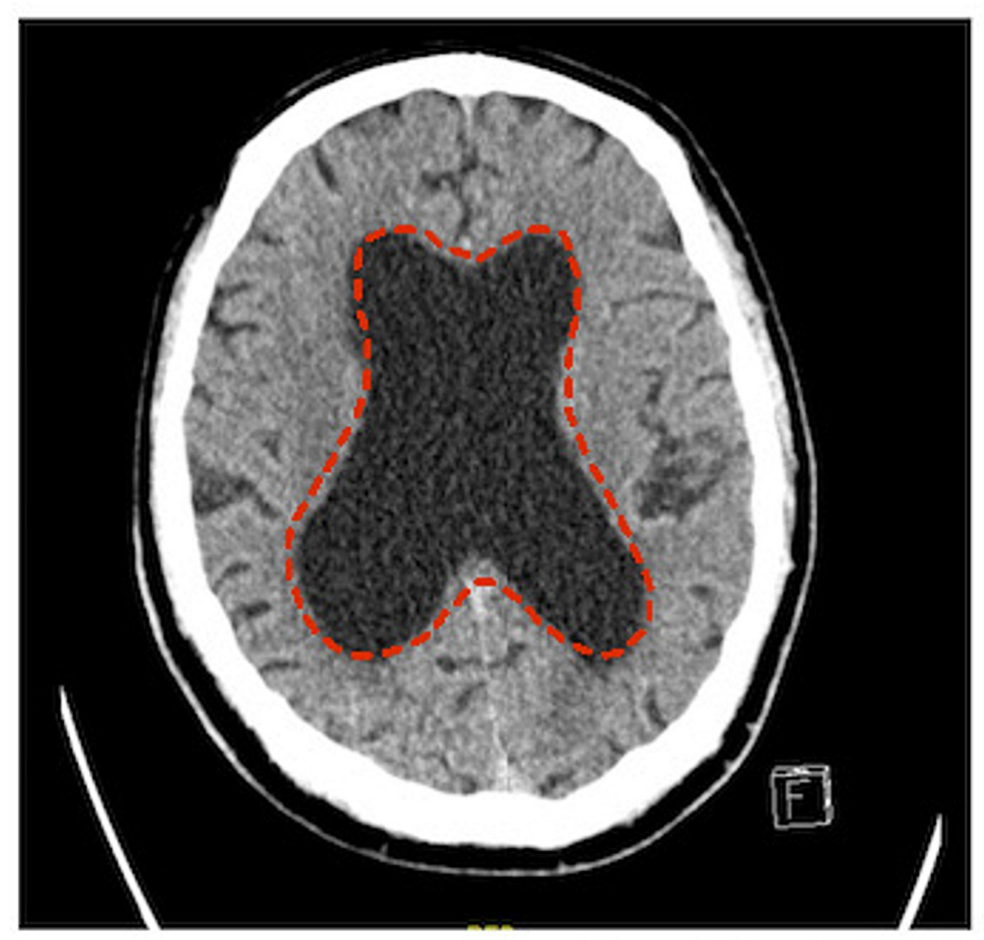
Cross-sectional computed tomography scan of the brain showing dilatation of the lateral ventricles (dashed border)
